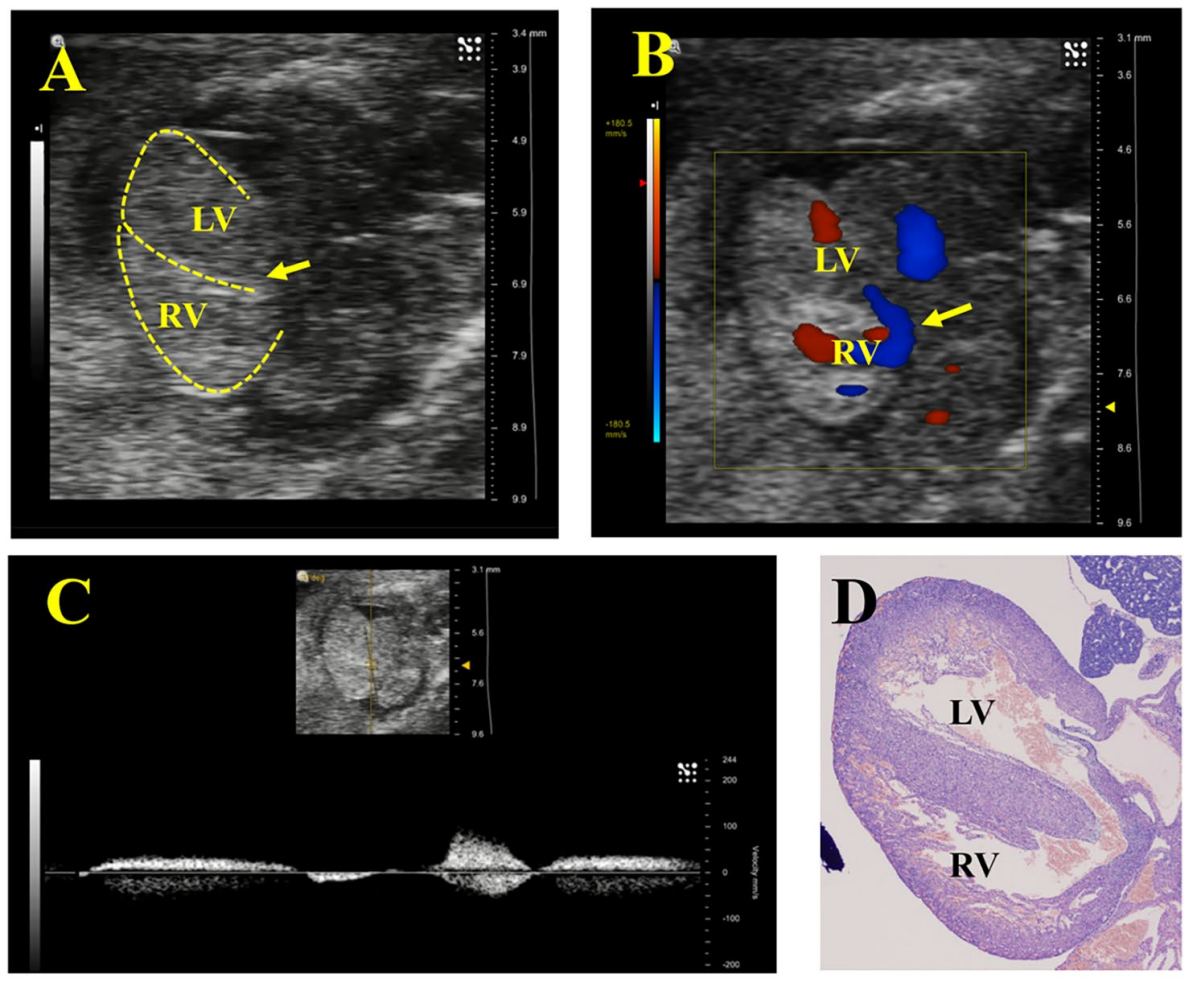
Prenatal cardiac ultrasound and pathological images of ventricular septal defect (VSD) fetuses. A is a B-mode image. B is a color Doppler image, showing transseptal blood flow. C is a pulse-wave Doppler mode, with detectable bidirectional blood flow at the ventricular septum. D is a pathological image (magnification is 40×), confirming a VSD. (The dotted line indicates the ventricular wall and septum, and the arrow points to VSD. LV, left ventricle; RV, right ventricle)
